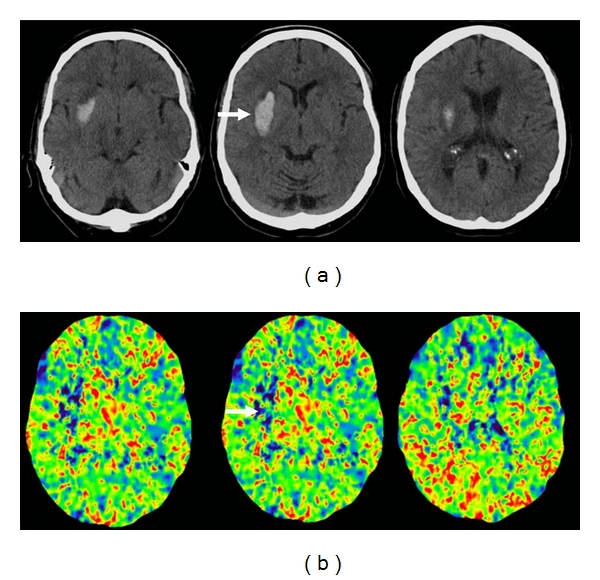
67-year-old man presented with left-sided hemiparesis. (a) Axial unenhanced CT images 2 hours after stroke show a hematoma lateral to the right lentiform nucleus with minimal surrounding hypodensity (arrow). The caudate nucleus is spared. (b) Similar to Figure 1, there is an area of decreased cerebral blood flow in the area of the hematoma. The overall picture is consistent with intracerebral hemorrhage.
